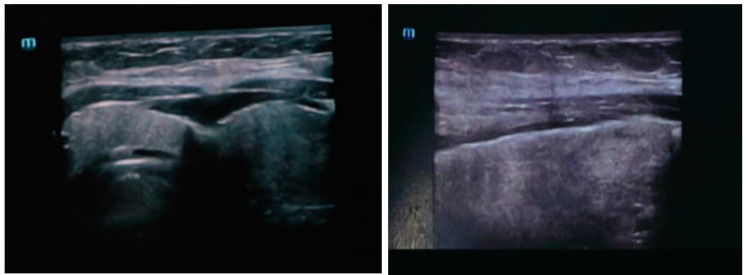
The ultrasound examination showed right parotid gland and right submandibular gland enlarged inhomogeneous echo structure with diffused increase in echogenicity of the glandular parenchyma. There was also a diffuse increase in intraparenchymal vascularity and reactive adenitis. No fluid collection, obstructing stones, dilatation of salivary duct, masses, or abscesses were observed.
